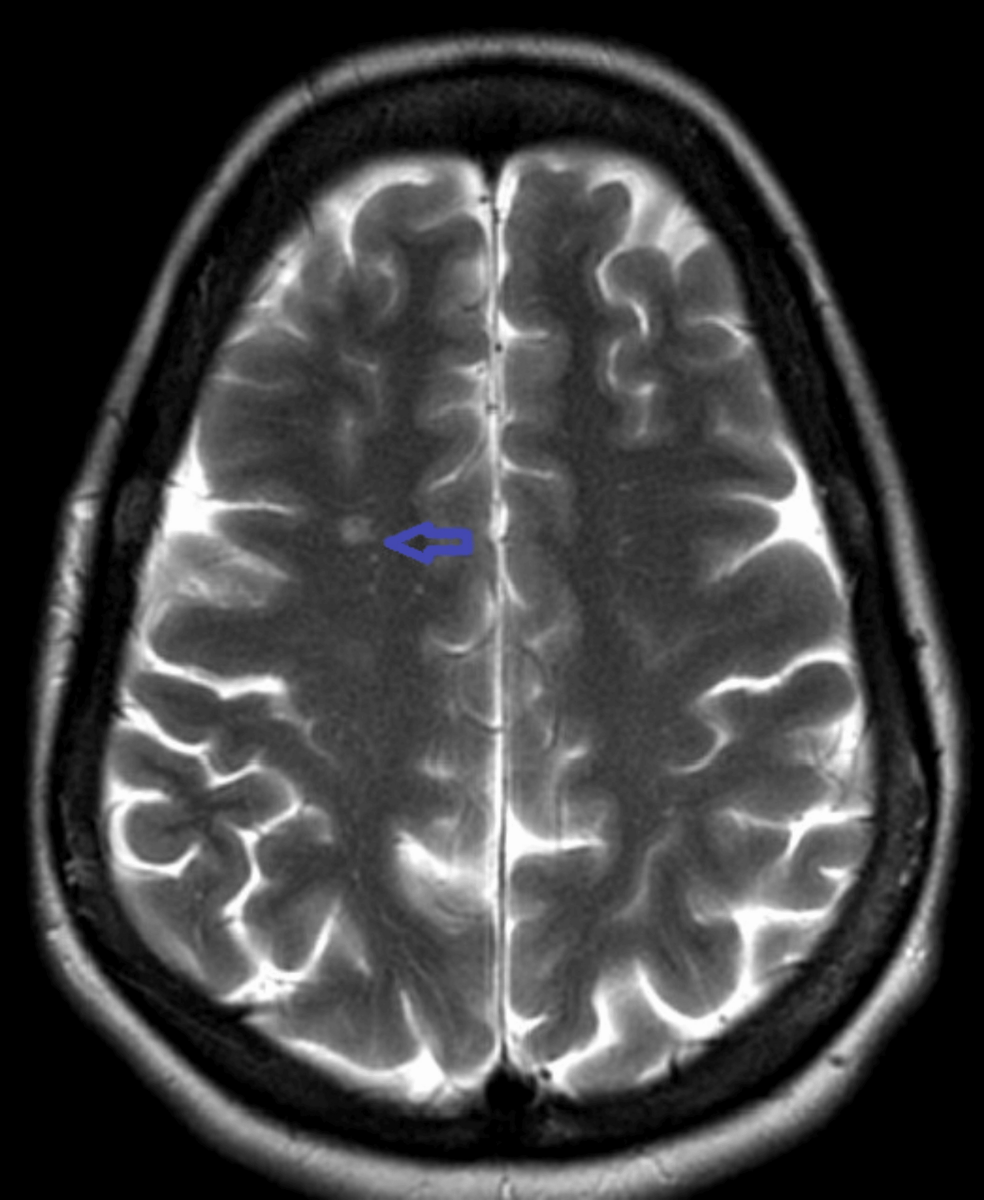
Axial brain MRI demonstrating a demyelinating lesion Axial T2-weighted MRI of the brain showing a small hyperintense subcortical white-matter lesion (arrow) consistent with demyelination.
